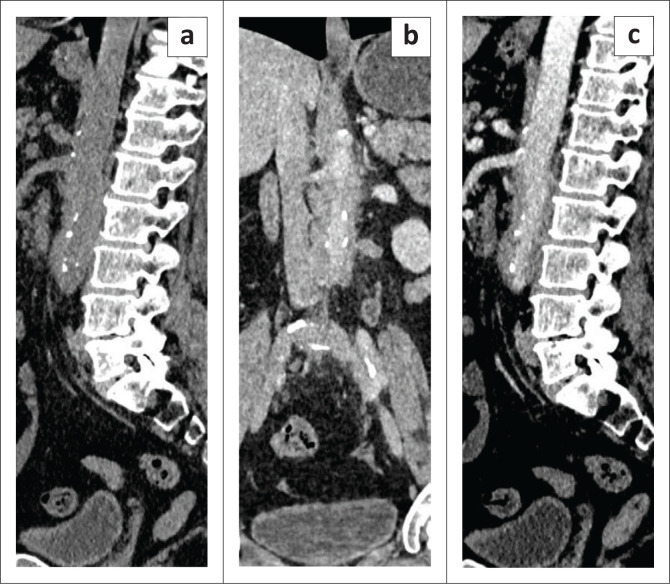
Sagittal (a and c) and coronal (b) sections of the CT abdomen indicate atheromatous calcific plaques on unenhanced CT (a) involving the aorta, with a soft tissue cuff involving the infrarenal abdominal aorta on contrast-enhanced CT (b–c).
